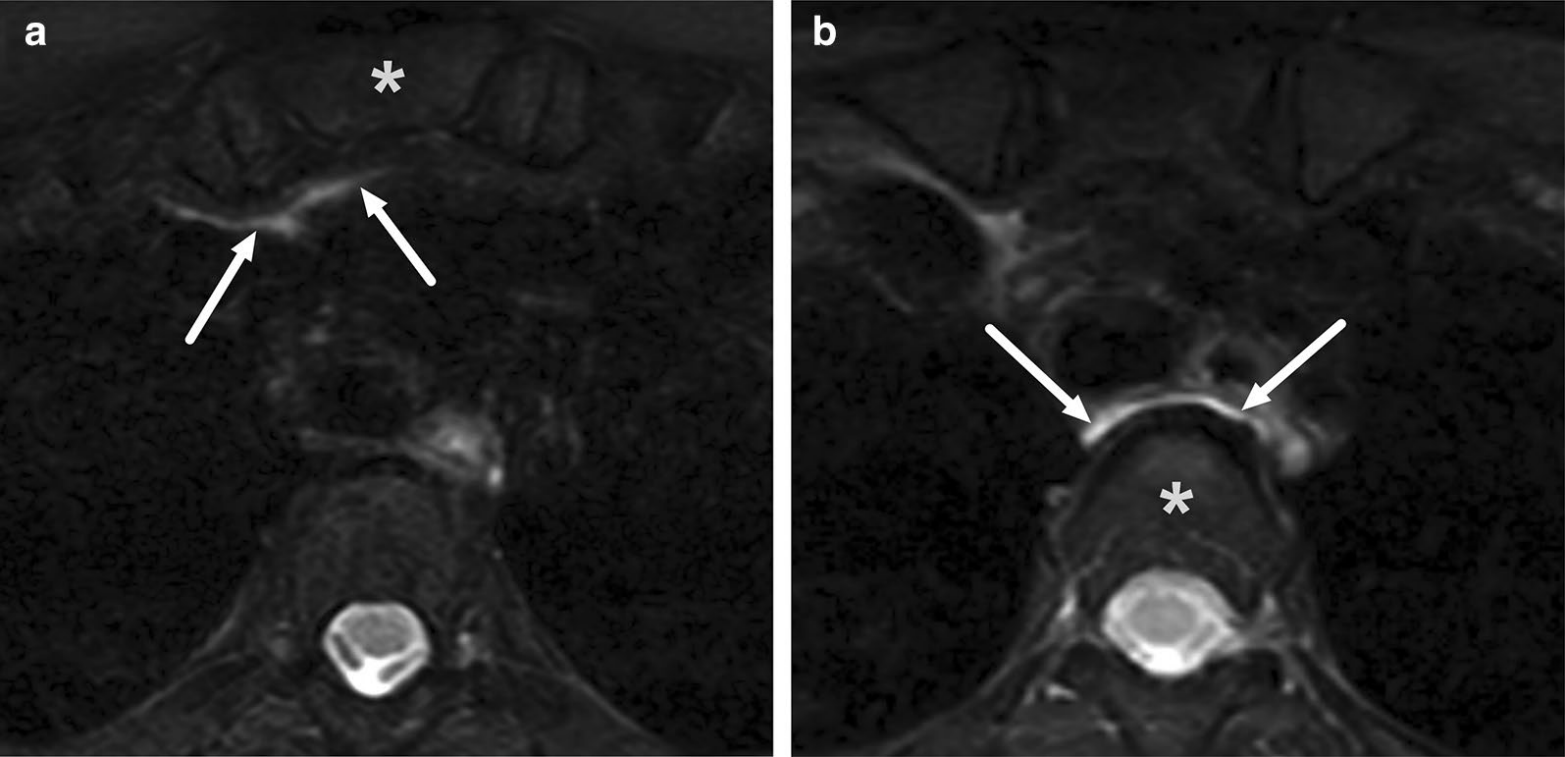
Examples of ME (arrows) on axial fat-suppressed T2-weighted images in two patients. Anterior ME (a) extends to the level of the sternum (asterisk), whereas posterior ME (b) is seen at or below the first thoracic vertebra (asterisk, in this patient the third thoracic vertebra). Image modified from ref. [12]
